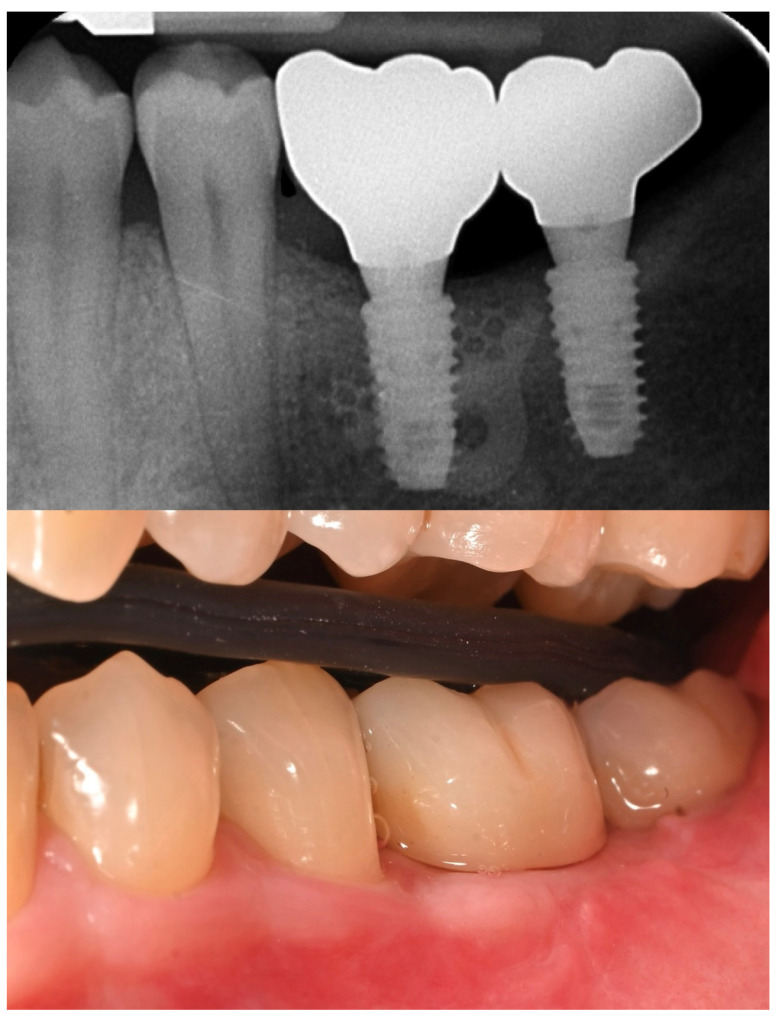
One-year radiographic and clinical follow-up.
